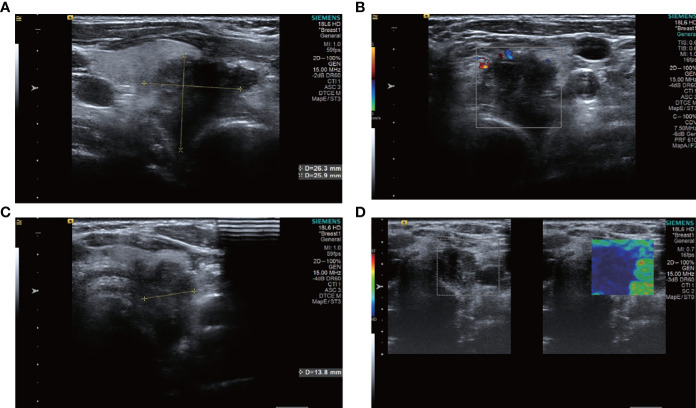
ultrasound images of patient #2, showing a hypoechoic nodule with indistinct borders in the left lobe of the thyroid gland, which was clearly visible as a nodule breaking through the thyroid peritoneum and invading the surrounding tissue. The patient underwent surgery only and had a survival period of 2 years and 7 months. (A) Sagittal scan (B) CDFI (C) Transverse scan (D) Elastography.
